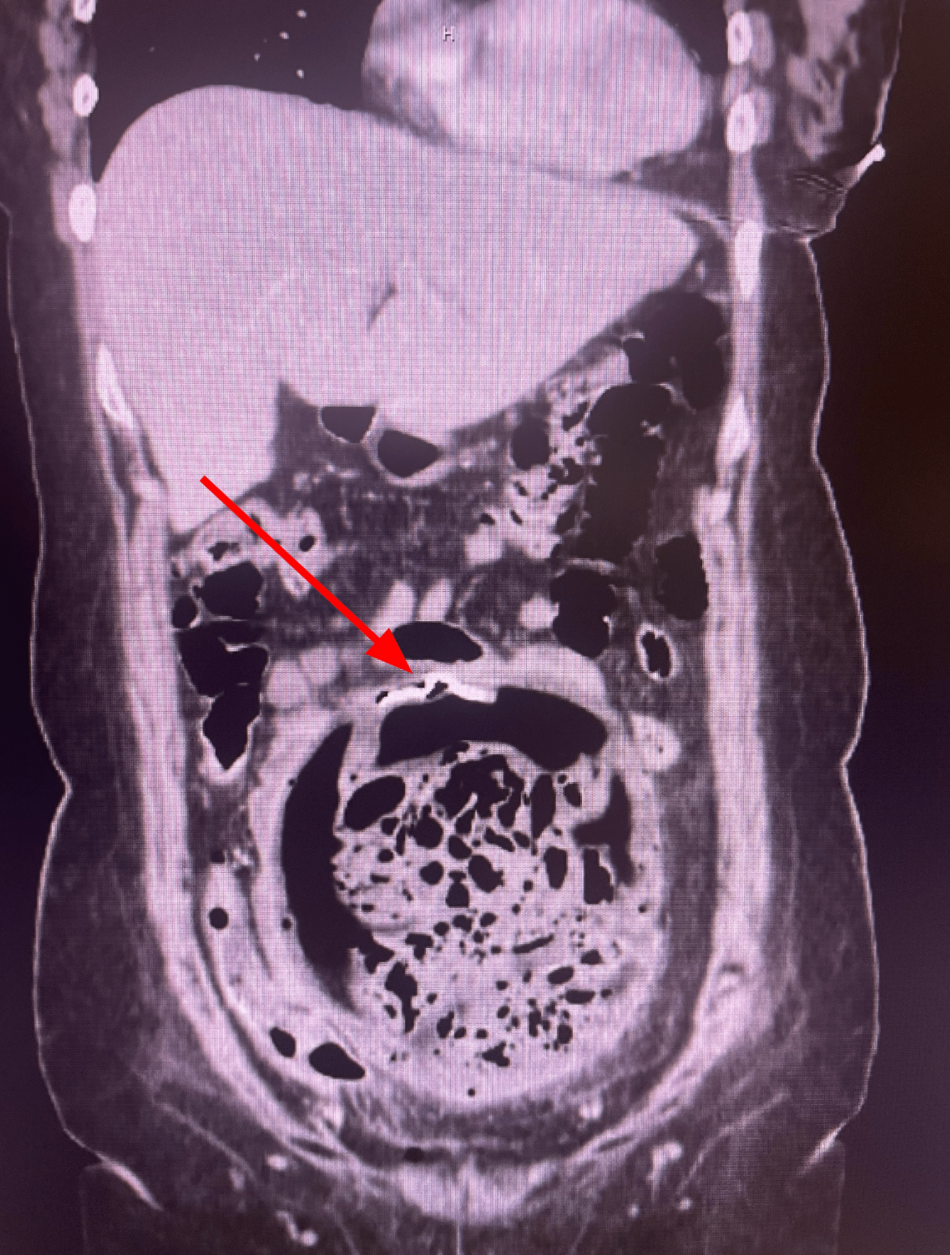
Coronal CT reveals a large, complex intra-abdominal abscess measuring approximately 9.5 x 15 x 16 cm, located in the mid-abdomen/pelvis region. A radiodense ribbon consistent with a retained surgical towel is visible within the abscess cavity, indicated by the red arrow
